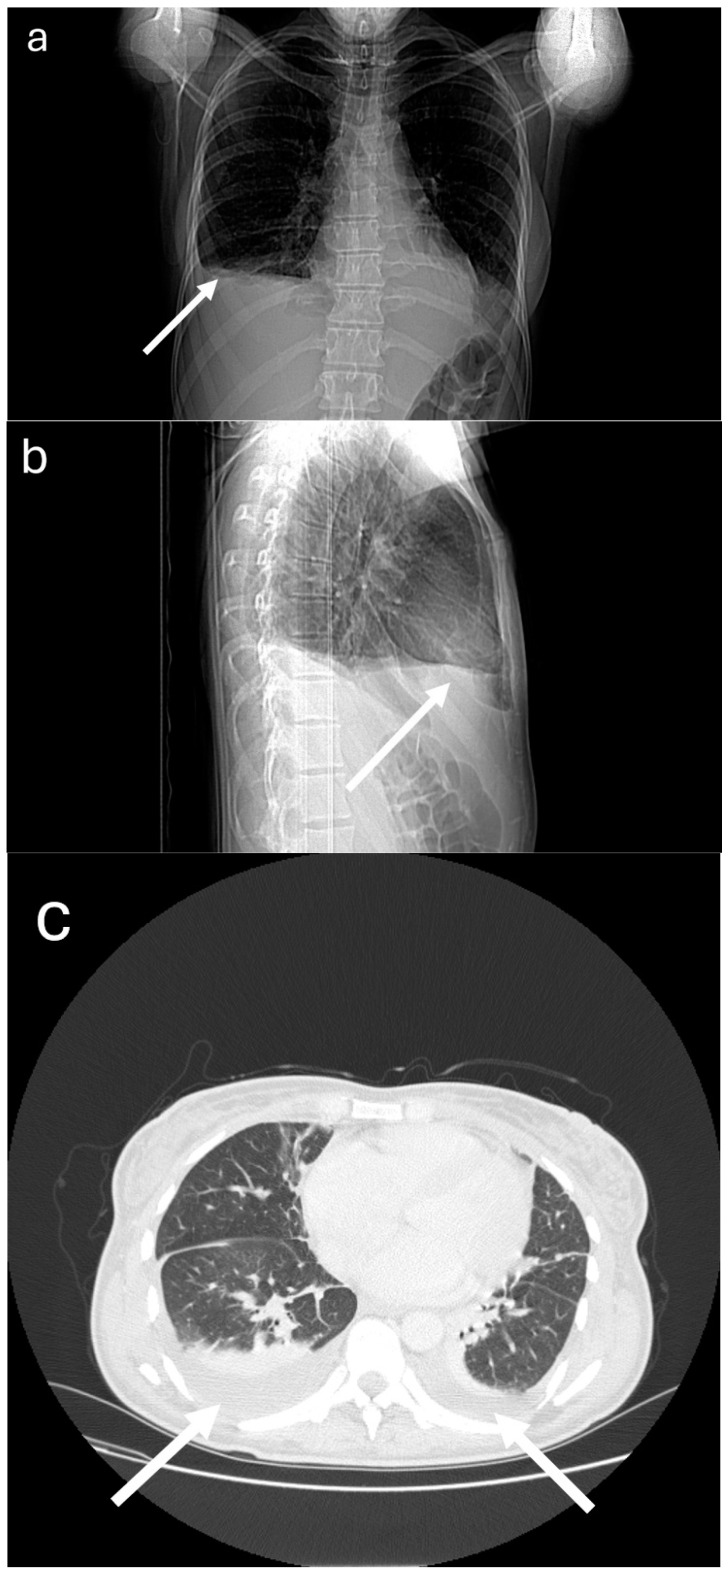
Patient’s chest CT examination: (a) frontal overview; (b) right sagittal overview; (c) axial scan; pleural effusion is indicated with an arrow.
